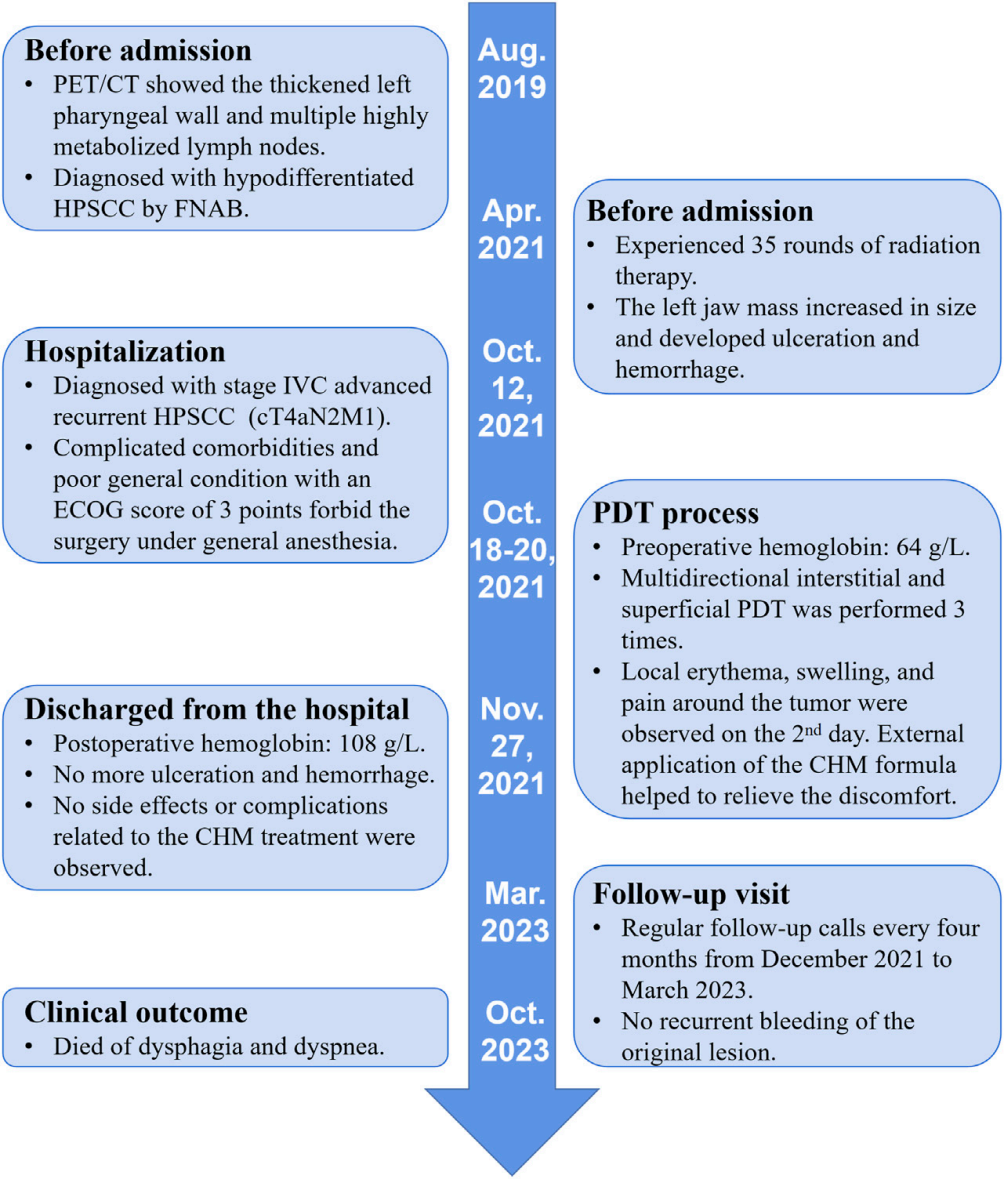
The timeline of this case. CHM, Chinese herbal medicine; CT, computerized tomography; FNAB, fine-needle aspiration biopsy; HPSCC, hypopharyngeal squamous cell carcinoma; PDT, photodynamic therapy.
